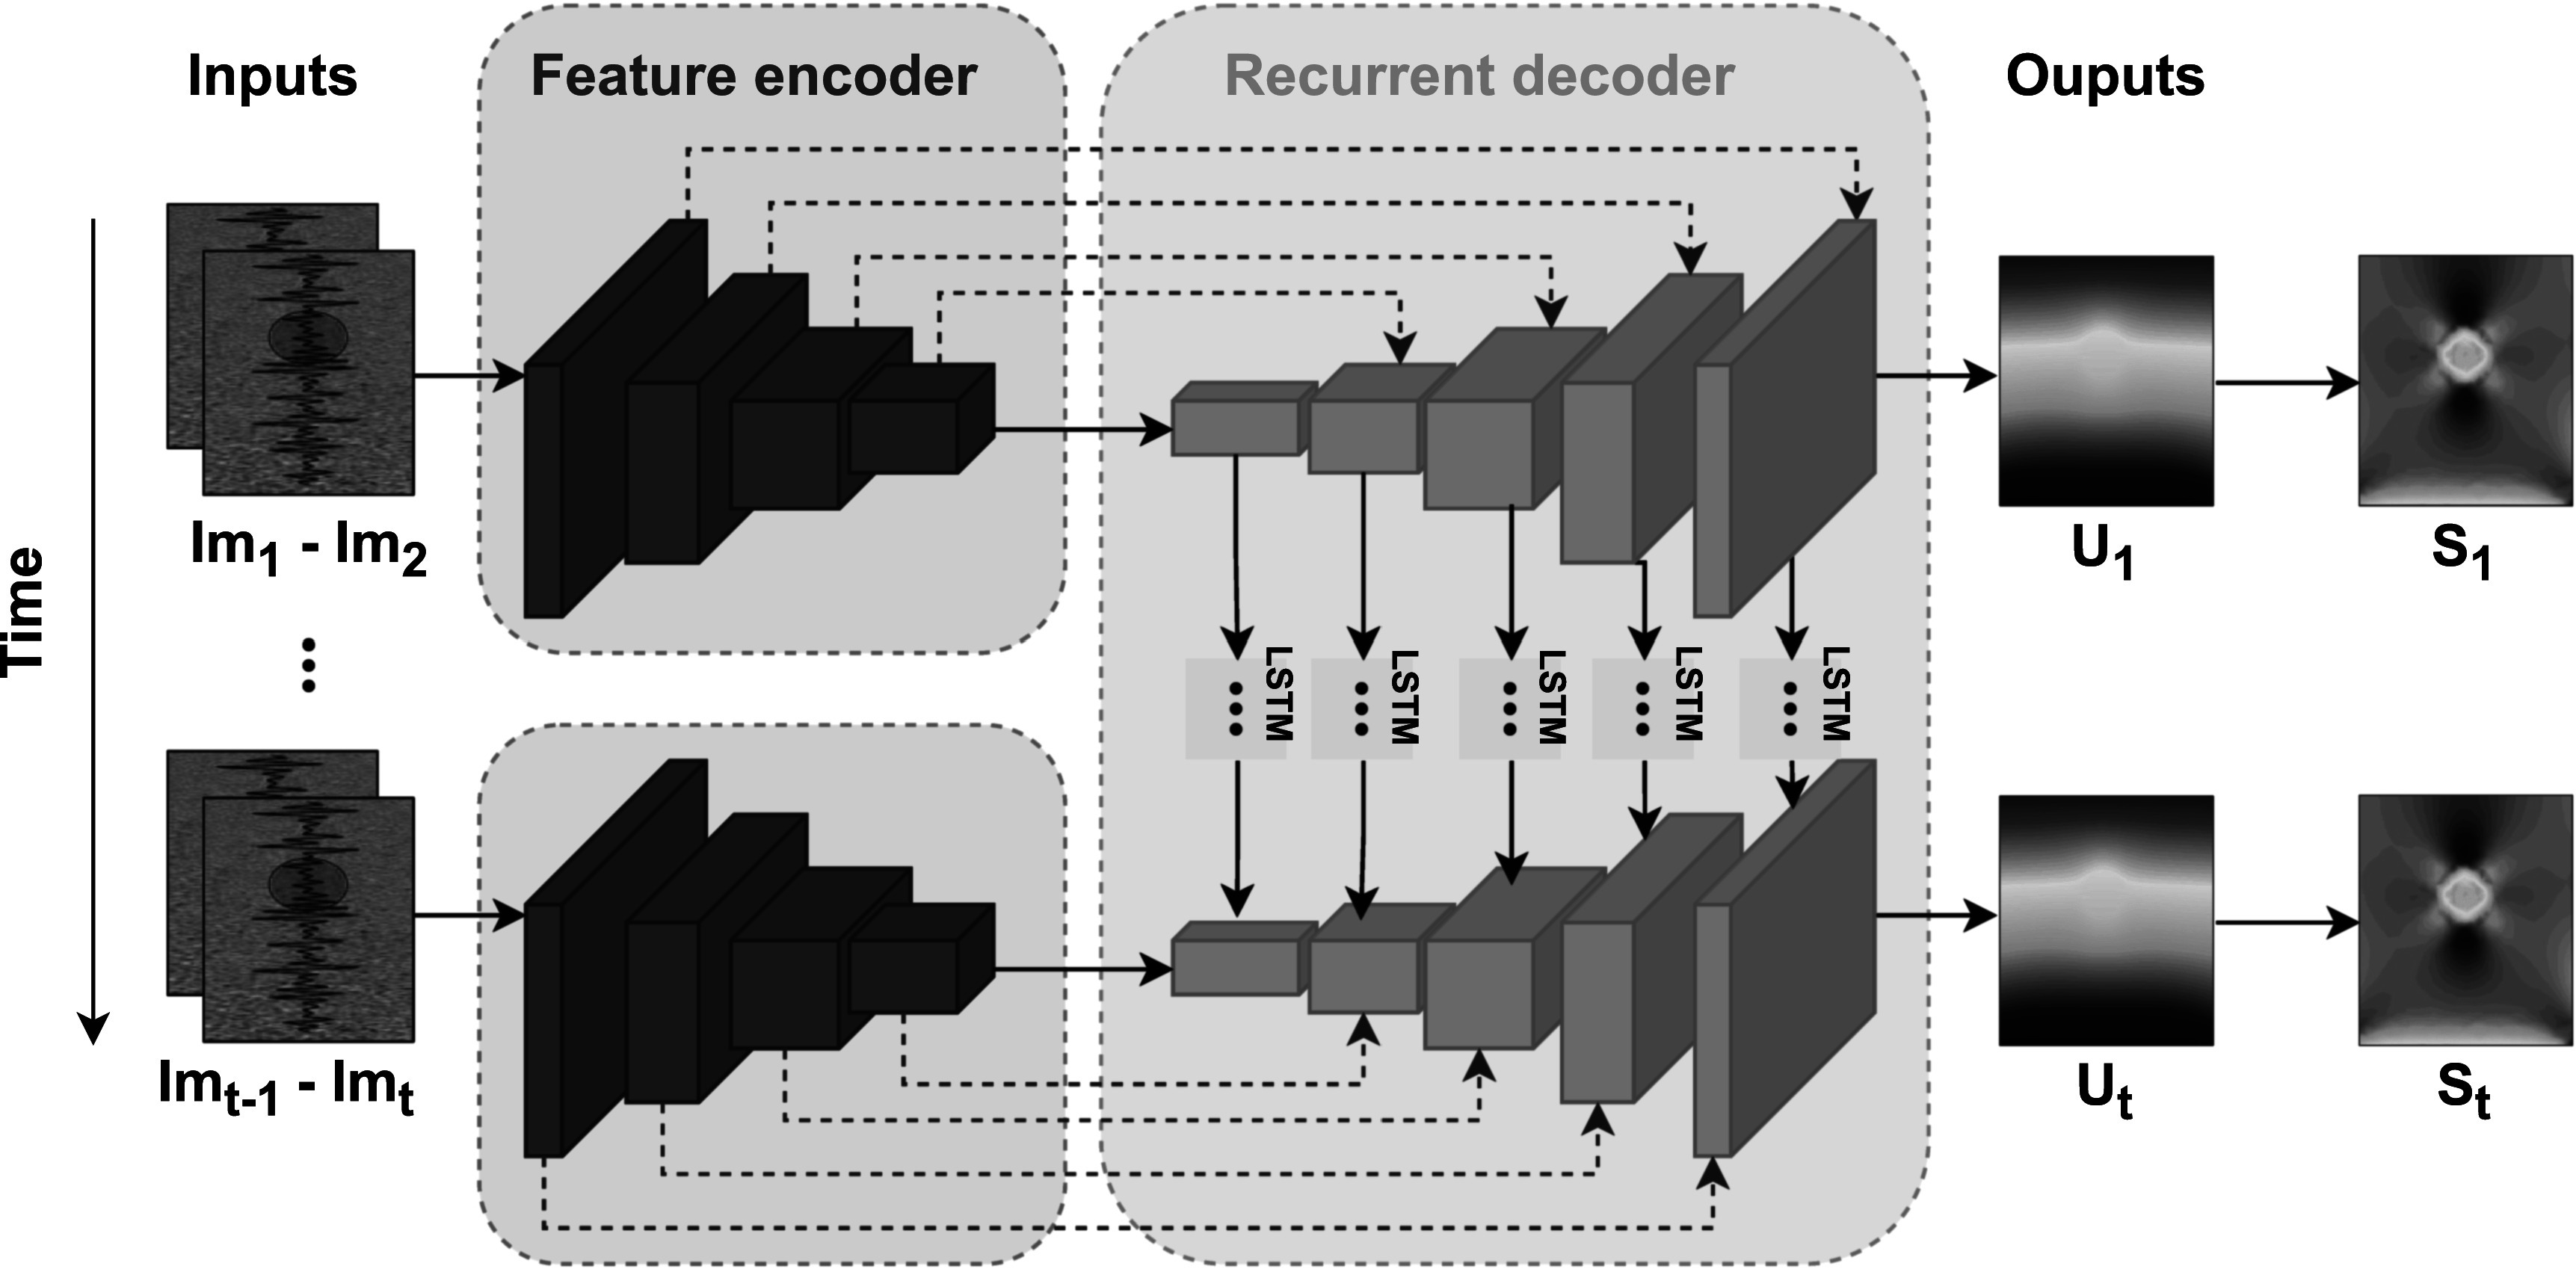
Overview of the proposed recurrent model for ultrasound strain elastography (ReUSENet). At each time step *t*, the network takes as inputs a pair of radio-frequency data frames (${{\mathrm{Im}}}_{t-1},{{\mathrm{Im}}}_{t}$) and outputs the dense displacement field *U*
